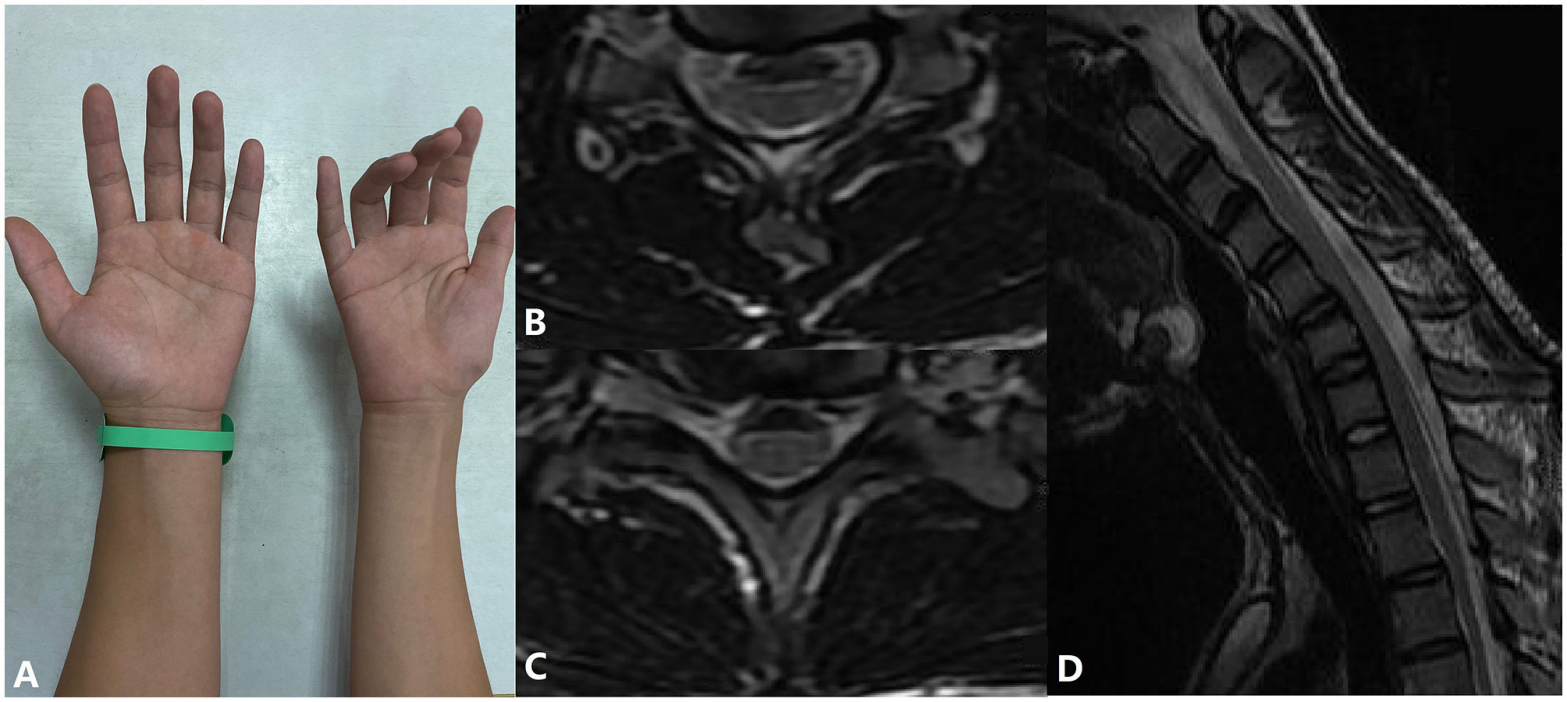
A patient with atrophy of the muscles of the right hand and forearm and his cervical flexion MRI. (A) Inability to extend fingers and muscle atrophy in the patient's right hand. (B,C) Spinal cord flattening and LOA in horizontal view. (D) LOA in sagittal view.
